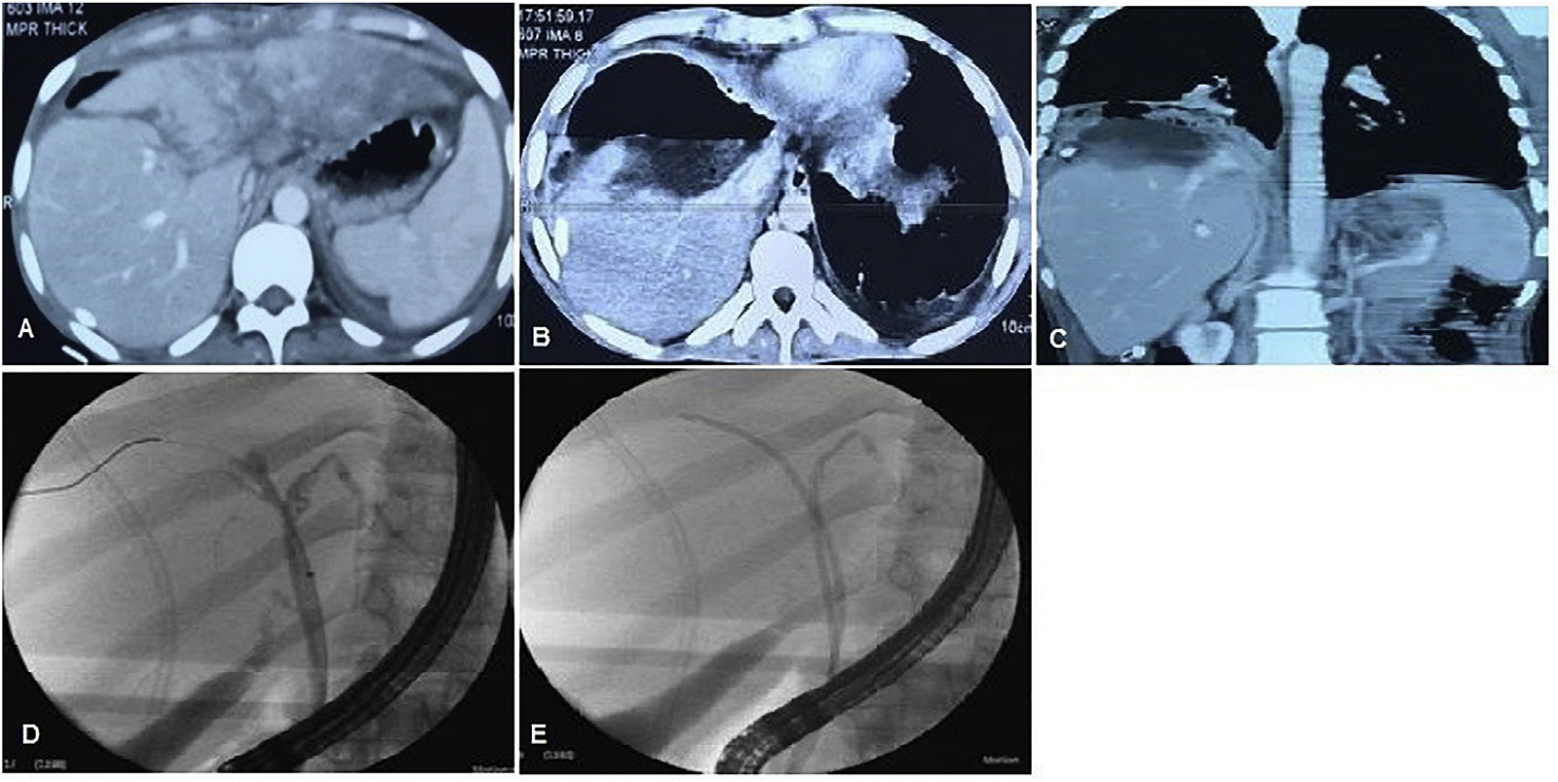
A) CT image of male patient, 20 years old, RTA blunt trauma, shows grade IV liver trauma, B,C) large fluid collection at site of Segments 4 & 8 with air fluid level due to liver abscess complicating central trauma with subsequent broncho-biliary fistula (BBF) & left lobe congestion. D) ERCP images for management of left BBF. E) Both the right and left biliary were cannulated and two 7F 15 cm plastic stents were applied and the patient improved with un eventful follow up course.
